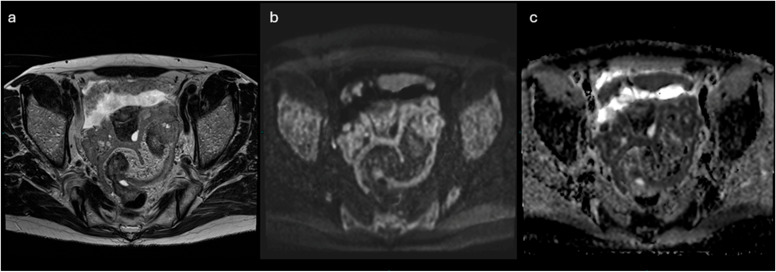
MRI scan of the pelvis of Patient of IMG. 1. The solid tissue in adnexal region shows intermediate signal intensity on T2-weighted sequences (a) and signal restriction on diffusion imaging (b,c), such as the solid implants along the peritoneum and the pelvic wall. See also the ascites in T2 sequences (a). Non-contrast MRI score: 5.
